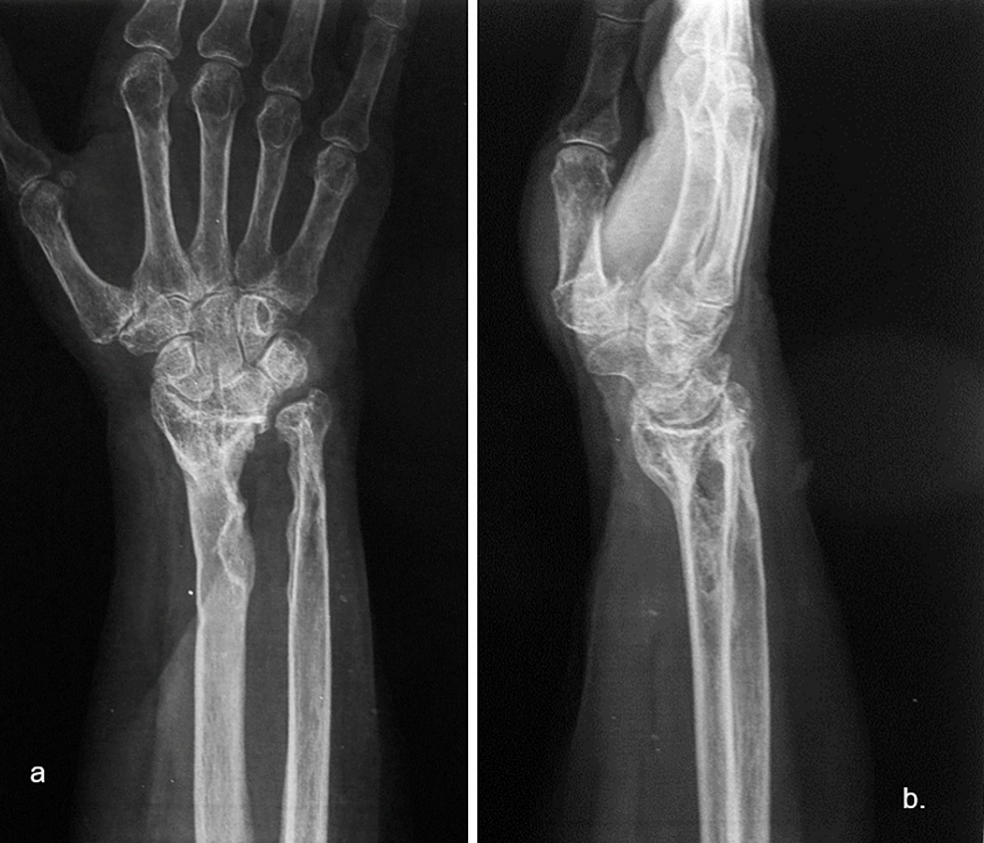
Postoperative X-rays images one year postoperatively. (a) Anteroposterior and (b) mediolateral.
